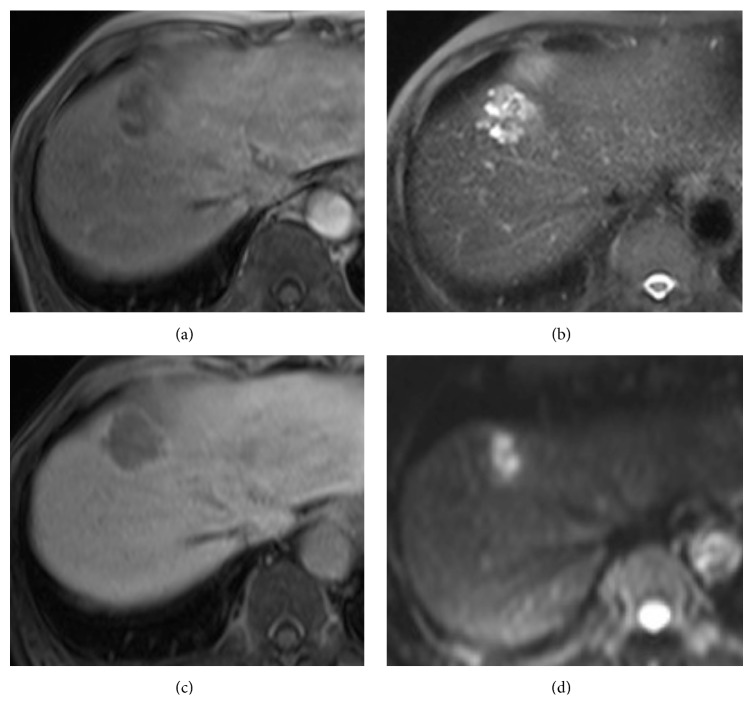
(a) T1-weighted magnetic resonance (MR) images showed the tumor with low intensity with lobulated borders. (b) The T2-weighted MR image shows the low signal portion indicating the cyst wall or bulkhead inside the high signal region. (c) In the hepatobiliary phase, the tumor showed low intensity. (d) On diffusion-weighted images, markedly high intensity was noted.
